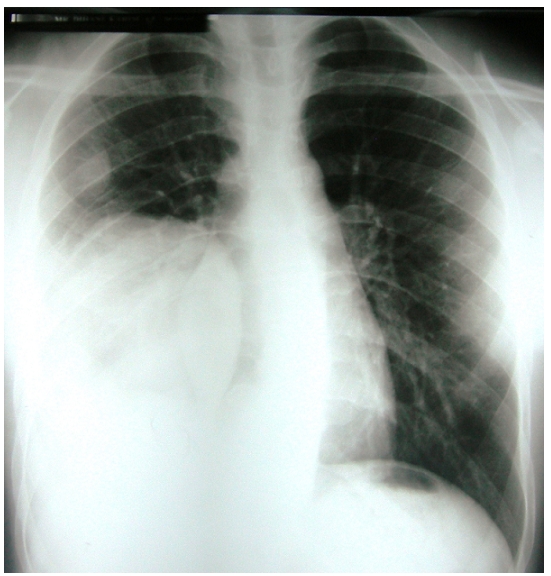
Radiographie thoracique de face montrant multiples opacités de tonalité
